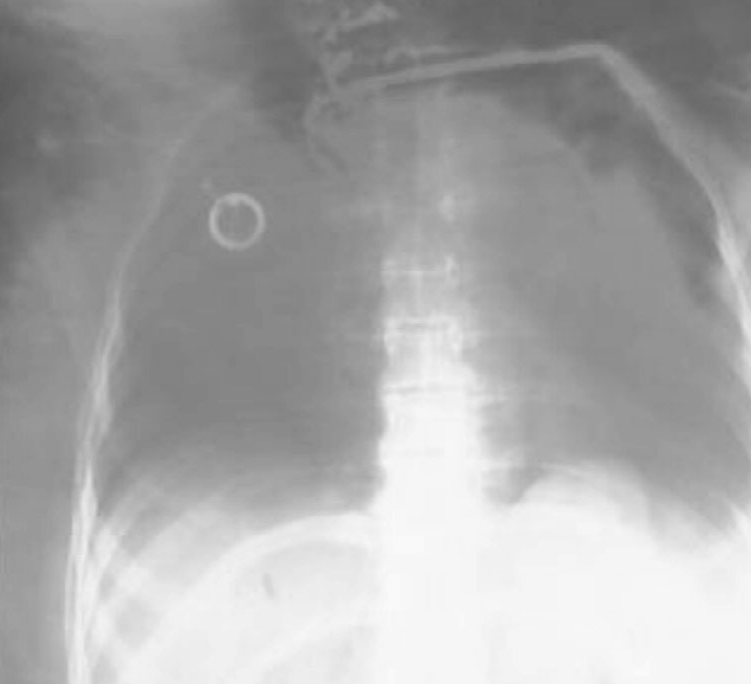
X-ray showing the intravascular catheter being fluoroscopically removed by means of a special endo-vessel grasper.
